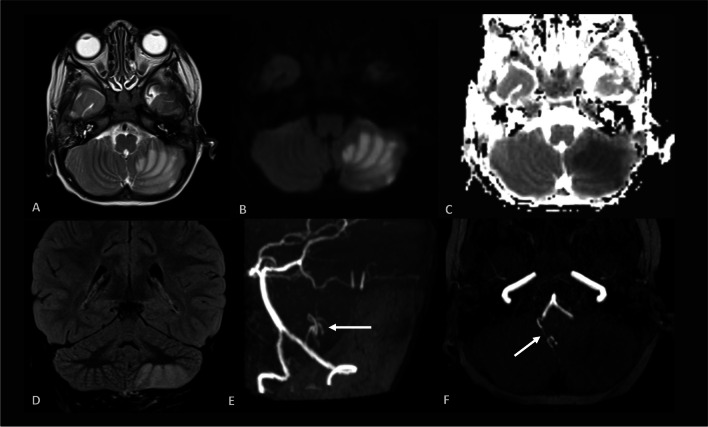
MRI brain scan showing left PICA acute ischemic stroke. The ischaemic area is hyperintense on axial T2 weighted images (A), shows diffusion restriction on axial diffusion weighted images (B) and apparent coefficient diffusion maps (C) and is hyperintense in coronal FLAIR (D). Magnetic resonance angiography, 3D maximum intensity projection (MIP) and axial MIP (E and F) show presence of the right PICA only (arrow) without visualisation of the left one
